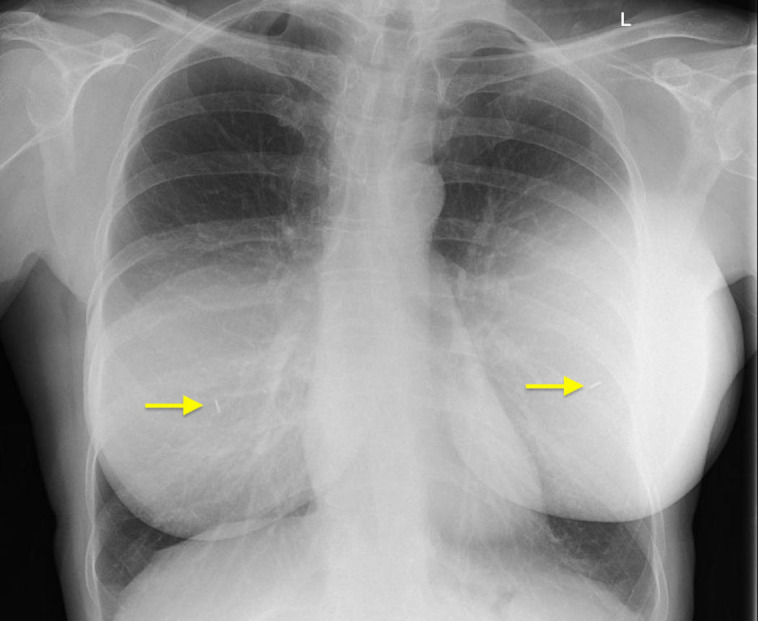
Radiofrequency chip visible on chest X-ray (highlighted by arrows).
